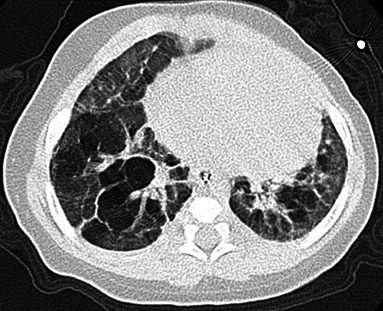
Axial CT on the 222nd DOL of case 1.
